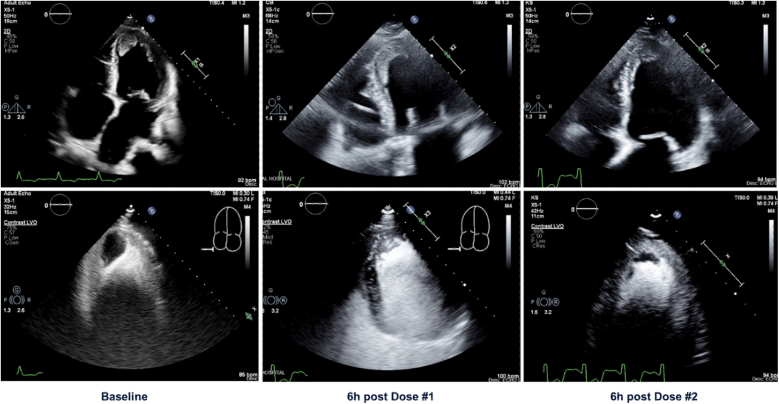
Echocardiographic Projections With and Without Contrast Highlighting Thrombus Size at Baseline (2.8 × 2.6 cm) and After the First (3.4 × 1.02 cm) and Second (2.03 × 0.8 cm) 25 mg tPA Infusions The third dose of tissue plasminogen activator (tPA) was stopped early due to right upper extremity hematoma, and an additional echocardiogram was not obtained.
